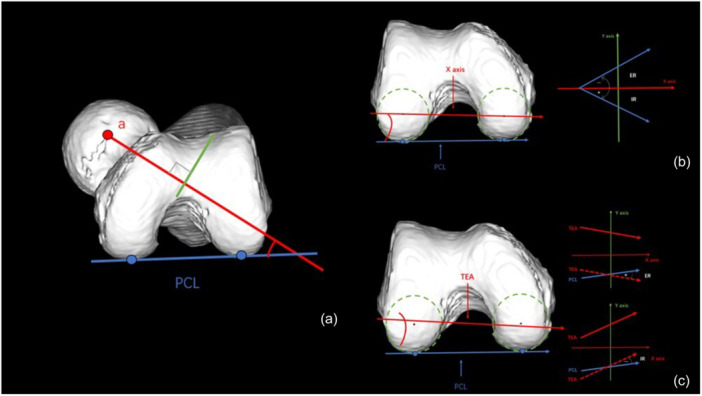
Measurement of femoral parameters. (a) Femoral neck anteversion was defined as the angle between a line (a) drawn perpendicular to the femoral shaft axis and the posterior condylar line (PCL), both projected onto the femoral XY plane. (b) The posterior condylar angle (PCA) was defined as the angle between the PCL and the femoral x‐axis in the XY plane, recorded as negative for internal rotation and positive for external rotation. (c) The condylar twist angle (CTA) was defined as the angle between the transepicondylar axis (TEA) and the PCL projected in the femoral XY plane.
